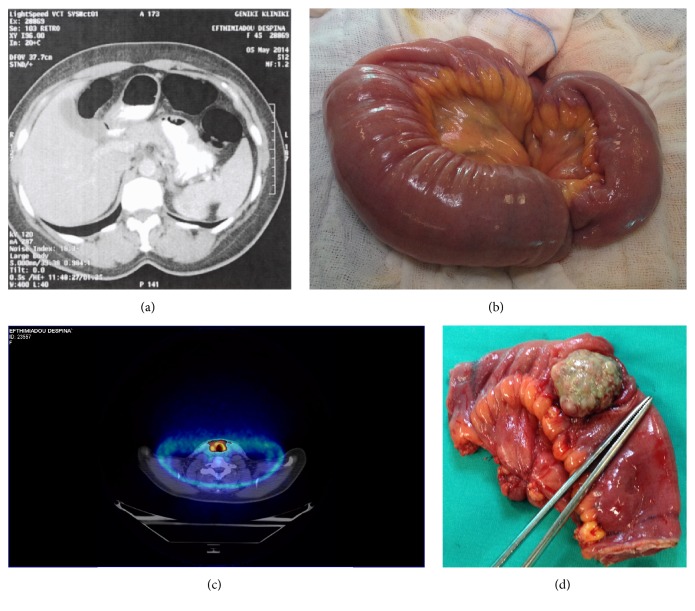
(a) CT scan showing an obstructing abdominal mass originating from the small bowel and causing intussusception; (b) intraoperative image of the intussusception; (c) thyroid gland with increased SUV in the 18FDG-PET/CT; (d) intestinal intussusceptions due to what appeared to be a distant melanoma metastasis.
